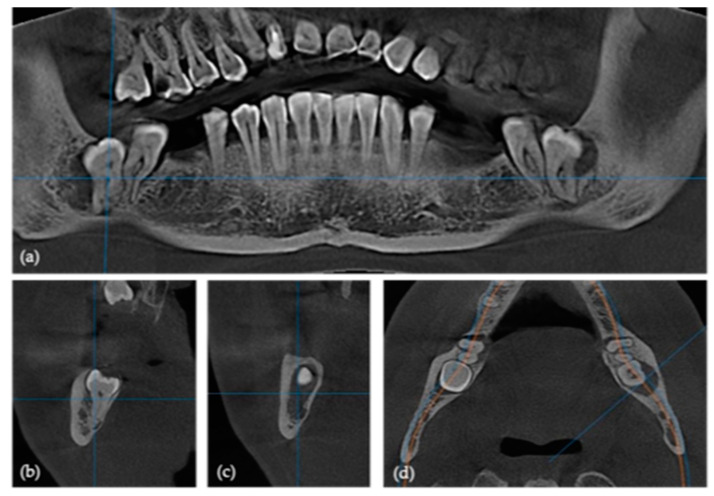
Preoperative CBCT images: (a) panoramic; (b,c) cross-sectional; and (d) axial views.
